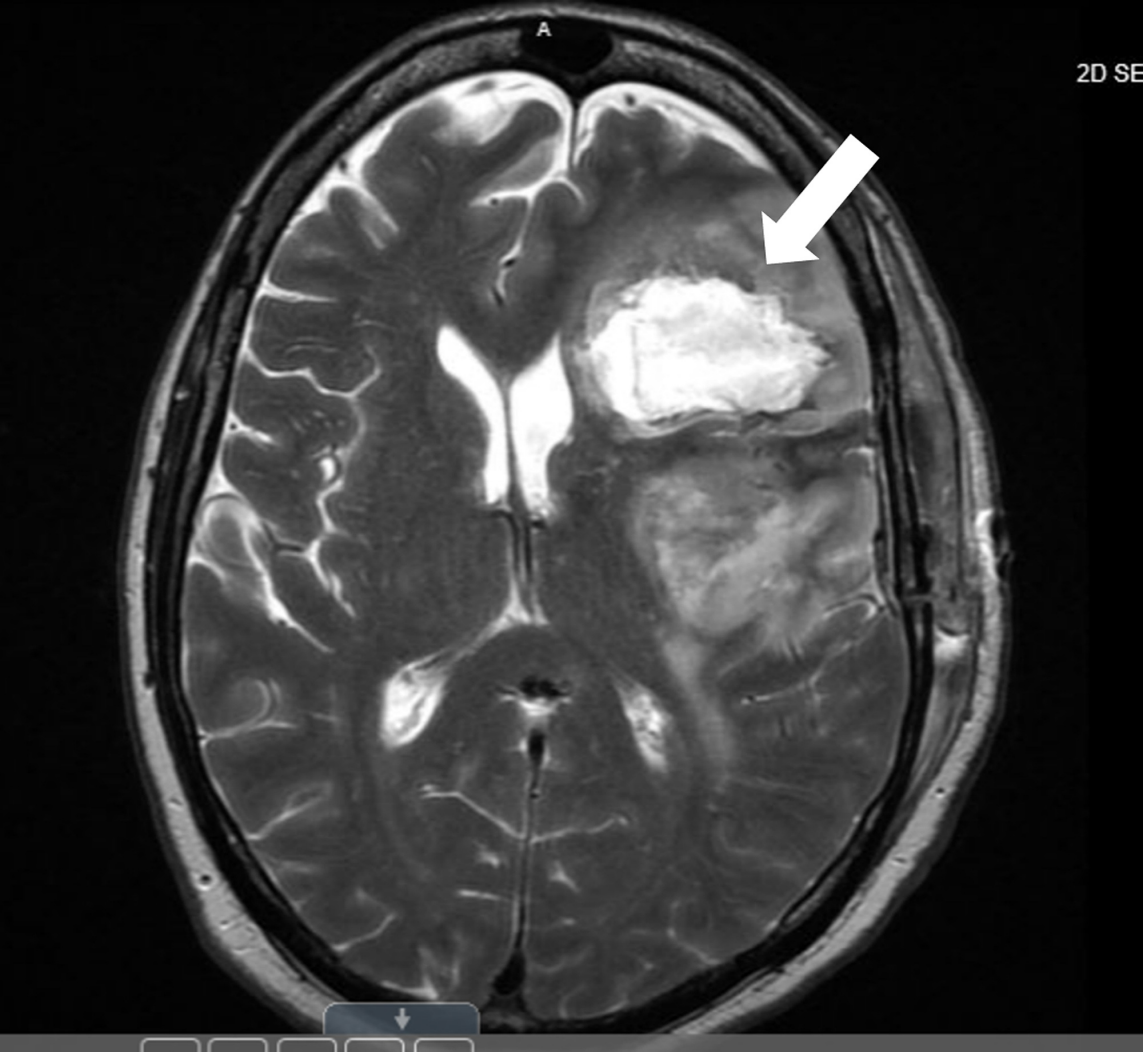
MRI of the brain reveals acute left posterior, frontal, and parietal infarct status post a recent partial resection of the left frontotemporal lobe tumor (indicated with a white arrow)
